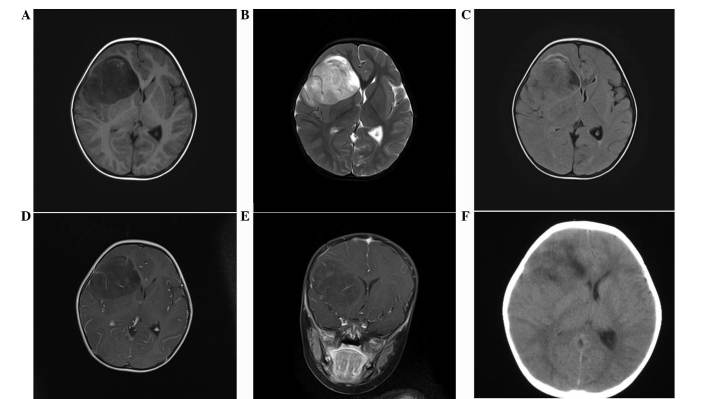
EVN identified in the right frontal cortex and subcortical white matter of a 2-year-old female. (A) T1-weighted image, (B) T2-weighted image, (C) FLAIR image and (D) axial and (E) coronal post-contrast T1-weighted image showing a moderate-to-marked diffuse and enhanced right frontal lobe lesion involving mainly the cortex and subcortical white matter. Peritumoral edema, cysts, hemorrhages and calcification were not observed. (F) CT scan revealed a large, microcystic and non-calcified right frontal lobe mass. EVN, extraventricular neurocytoma; FLAIR, fluid-attenuated inversion recovery.
